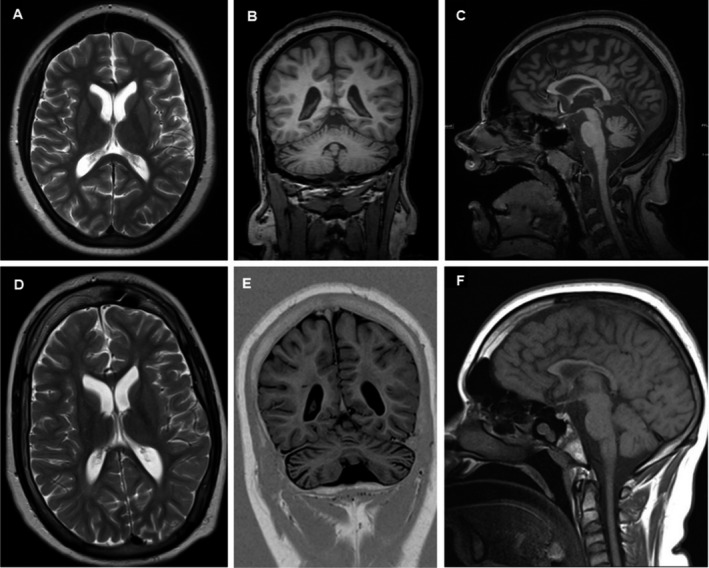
Brain MRI of individual II:1 (A–C) and individual II:2 (D–F). (A) Axial T2‐weighted, (B) coronal T1‐weighted TFE and (C) sagittal T1‐weighted TFE MRIs demonstrating supra‐ and infratentorial atrophy and hyperostosis of the skull. (D) Axial T2‐weighted, (E) coronal T1‐weighted inversion recovery, and (F) sagittal T1‐weighted SE MRIs demonstrating supra‐ and infratentorial atrophy, a thin corpus callosum and hyperostosis of the skull.
